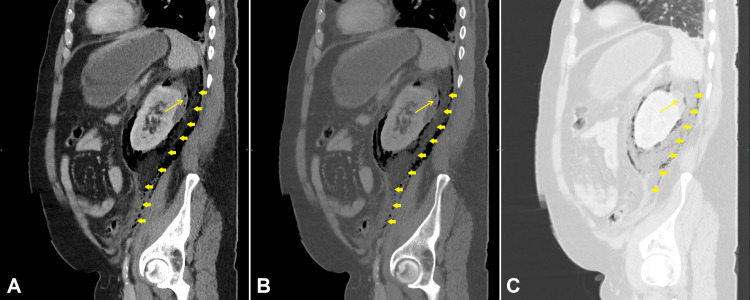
Sagittal reconstructions of enhanced abdominal CT in soft tissue (A), bone (B), and lung (C) windows centered on the left lung Subcapsular fluid is seen in the posterosuperior aspect of the left upper renal pole (Thin arrows) with a large crescent-shaped bubbly perinephric collection extending downwards in the retroperitoneal space along the psoas muscle, reaching the presacral space (Thick arrows). Thickening of Gerota's fascia is seen, which is consistent with emphysematous pyelonephritis.
